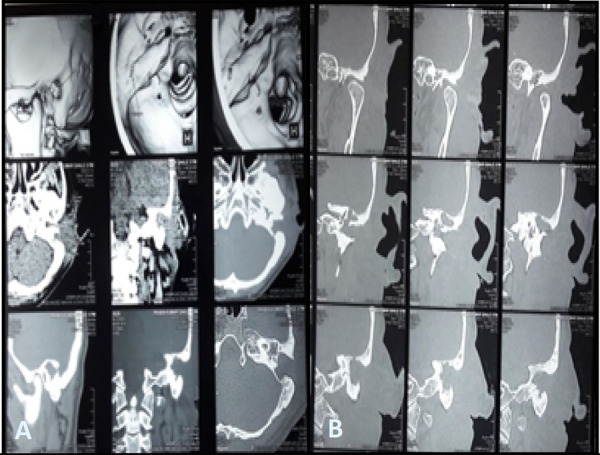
A,B. HRCT scan of temporal bone shows mastoid fistula and middle ear tumor.
